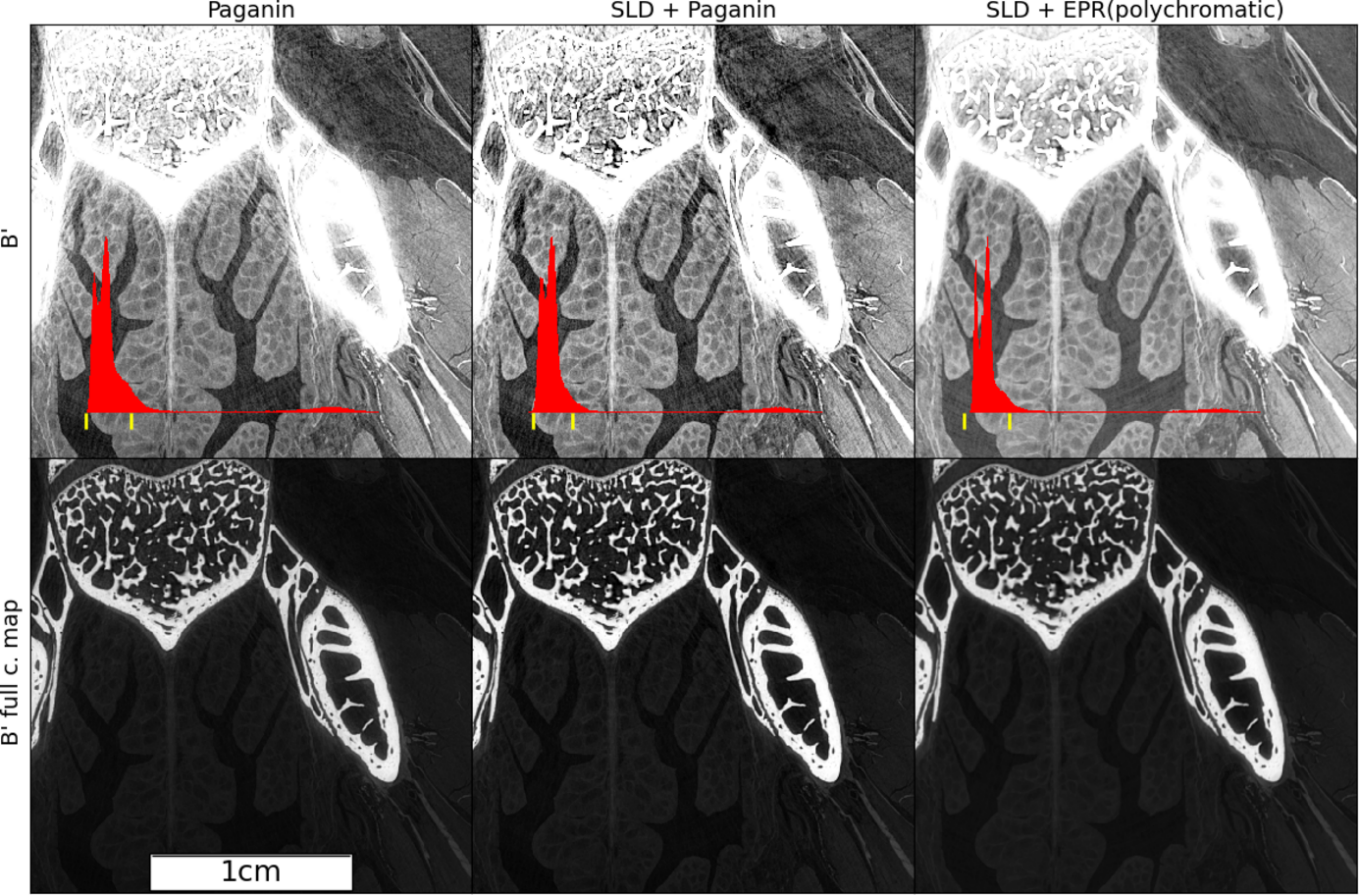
Incremental application of SLD and EPR: (left) reconstruction by the standard Paganin *et al.* algorithm, (center) the result by preprocessing the radiography with SLD, (right) the effect of both the SLD and EPR algorithms. In the first row the gray scale is adapted to the soft matter (the range is marked by yellow ticks in the red histogram); in the second it covers the whole range.
